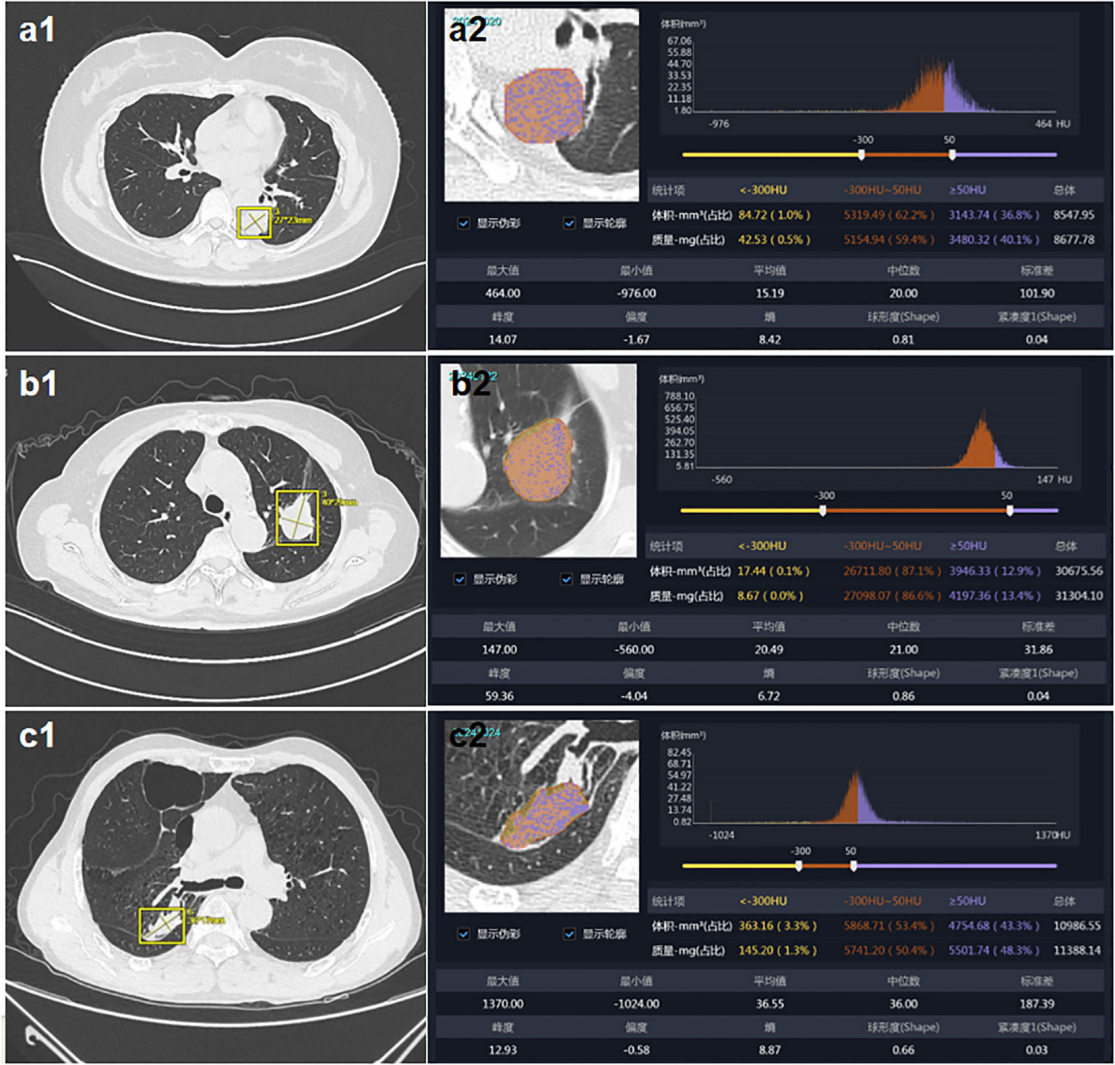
CT imaging features based on artificial intelligence analysis. (a) patient 1: female, age 40, tumor size is ≥3mm, with tumor dimensions of 27×23mm. (b) patient 2: female, age 68, tumor size is ≥3mm, with tumor dimensions of 40×29mm. (c) patient 3: male, age 66, tumor size is ≥3mm, with tumor dimensions of 36×17mm.
